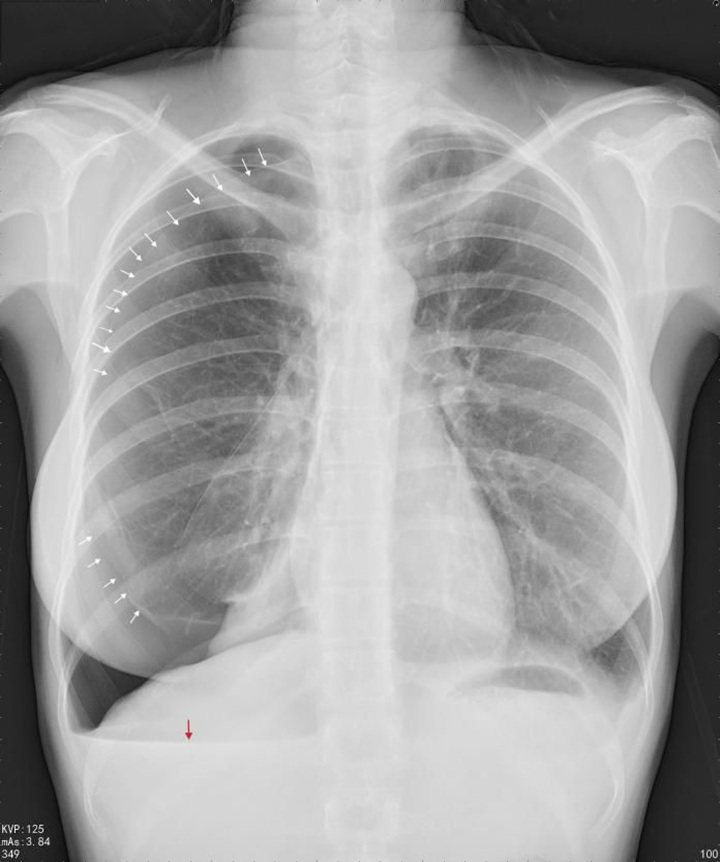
Chest radiograph captured during the patient’s first recurrence after surgery, with the white arrow indicating the visceral pleural line and the red arrow indicating the air-fluid level.
